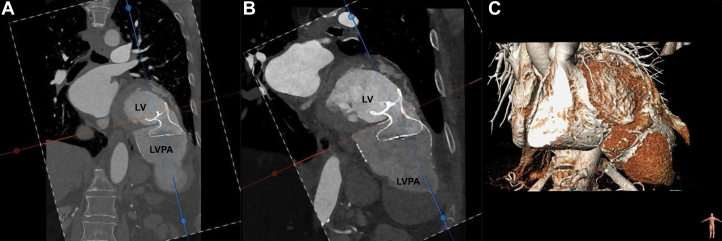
Coronal Cardiac CT (A) Postprocedural scan after percutaneous intervention. (B) Follow-up computed tomography (CT) scan. (C) Three-dimensional volume rendering reconstruction at follow-up. LV = left ventricle; LVPA = left ventricular pseudoaneurysm.
